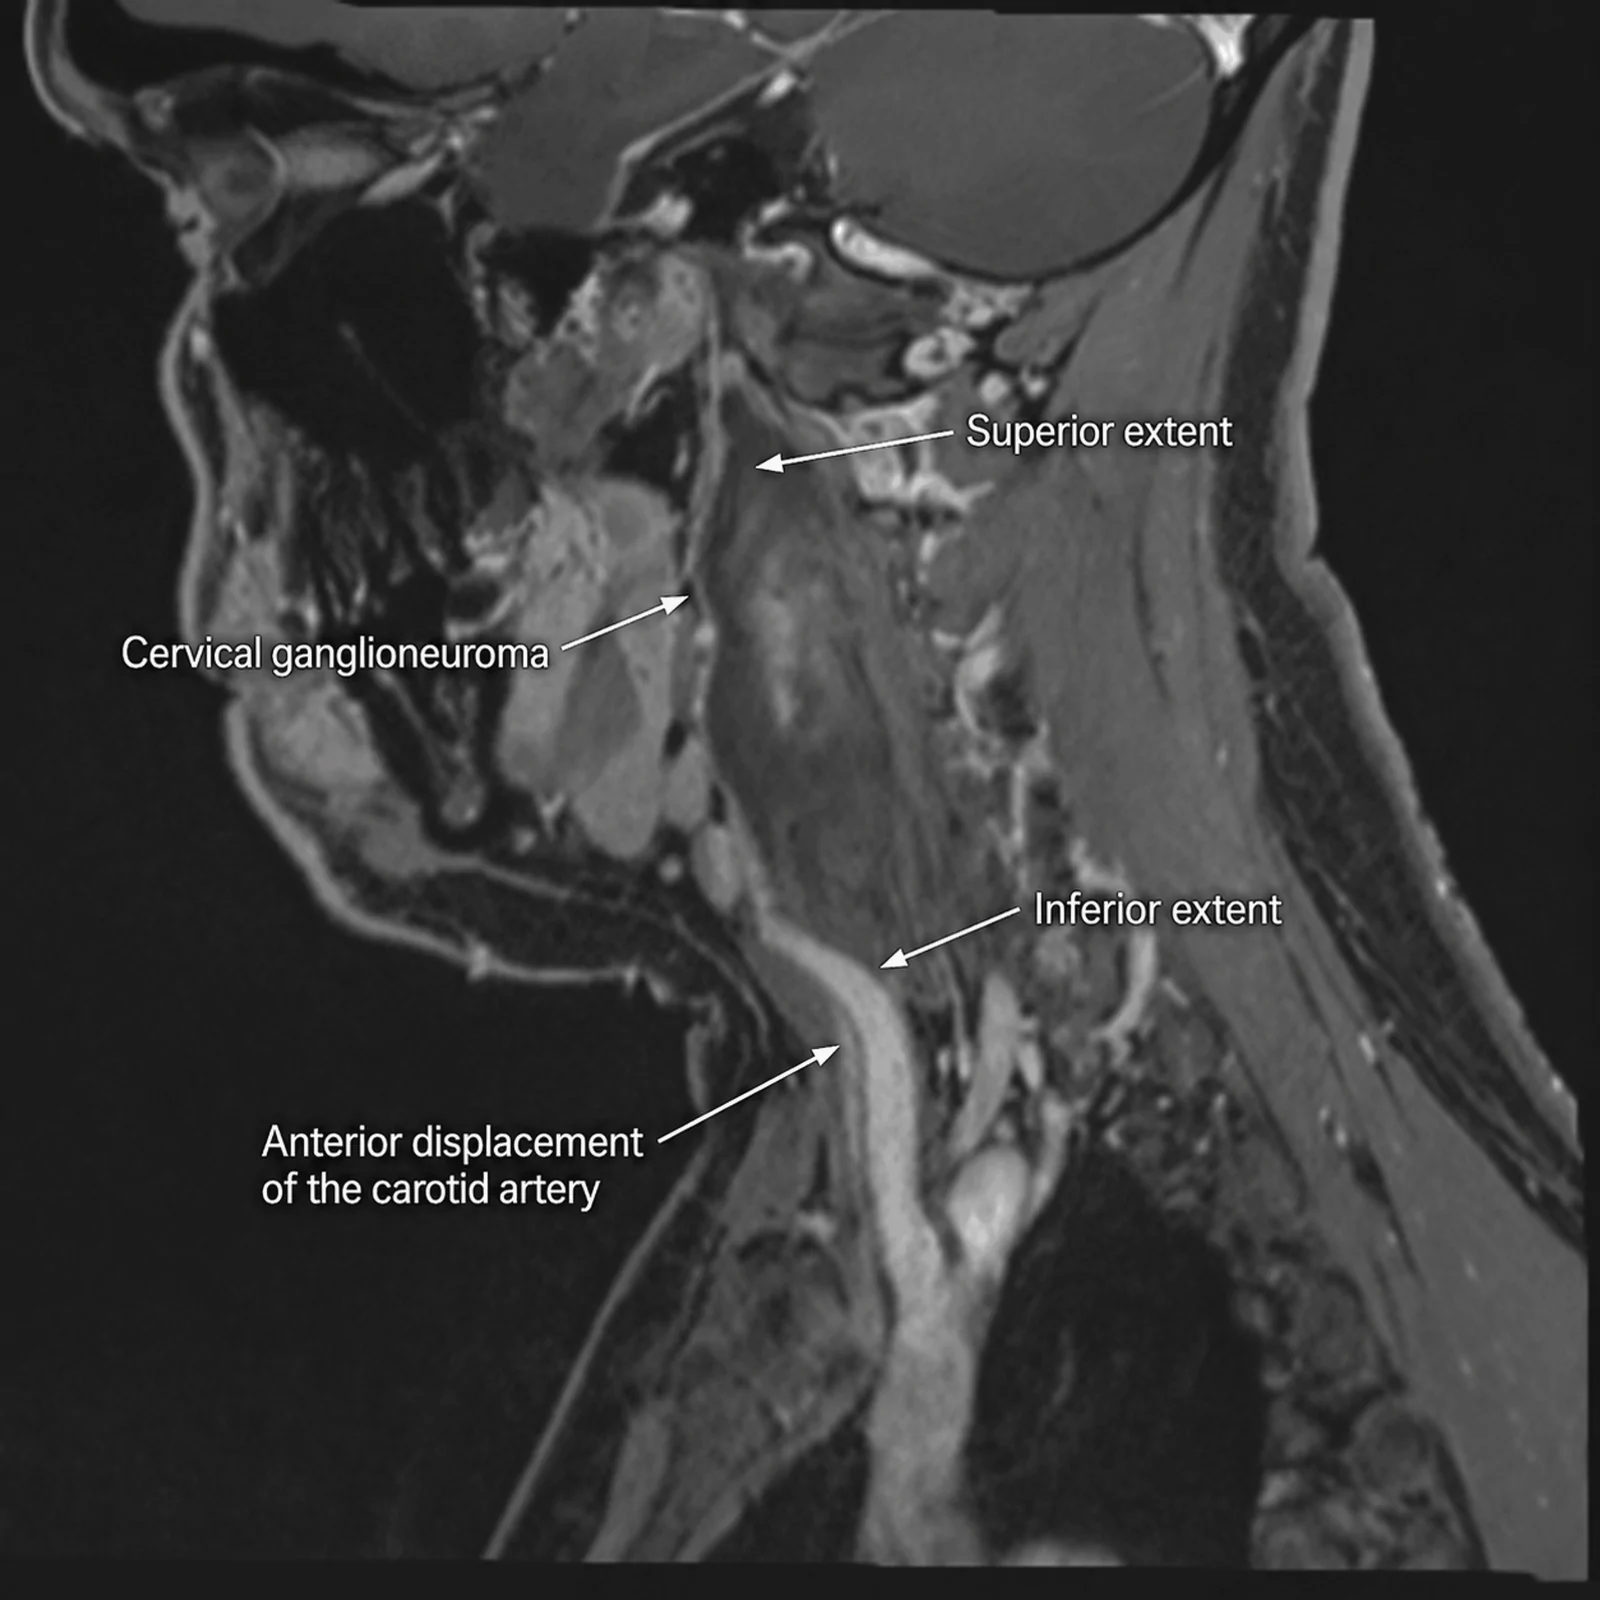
Sagittal contrast-enhanced T1-weighted MRI demonstrating a right carotid-space mass. Sagittal contrast-enhanced T1-weighted MRI of the neck demonstrates a large, sharply circumscribed fusiform mass within the right carotid space. The lesion extends from the level of the oropharynx at C1 to the glottis at C6 and measures 3.9 × 3.3 × 9.3 cm. Its elongated configuration and carotid-space location support a neurogenic origin. The arrow indicates the tumour.
